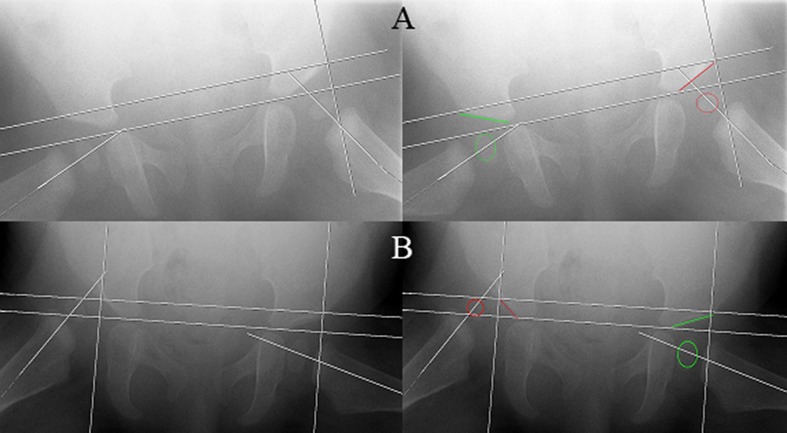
Frog-leg or Lauenstein AP radiograph. a The femoral head is positioned centrally within the true acetabulum with the hips flexed and abducted; this hip is regarded as subluxed as the femoral neck is not directed towards the triangular cartilage. b With hips flexed and abducted the femoral head remains positioned lateral to Perkin’s line and superior to the acetabular rim; this hip is regarded as dislocated
